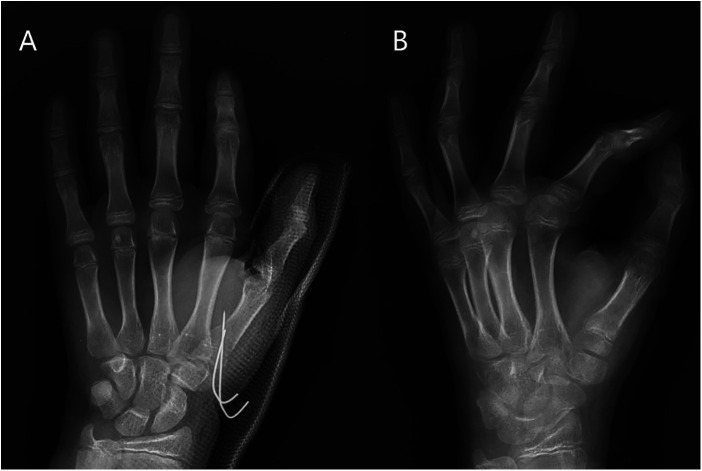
(A) Result after closed reduction. (B) 8 weeks follow-up x-ray after closed reduction with pinning.
